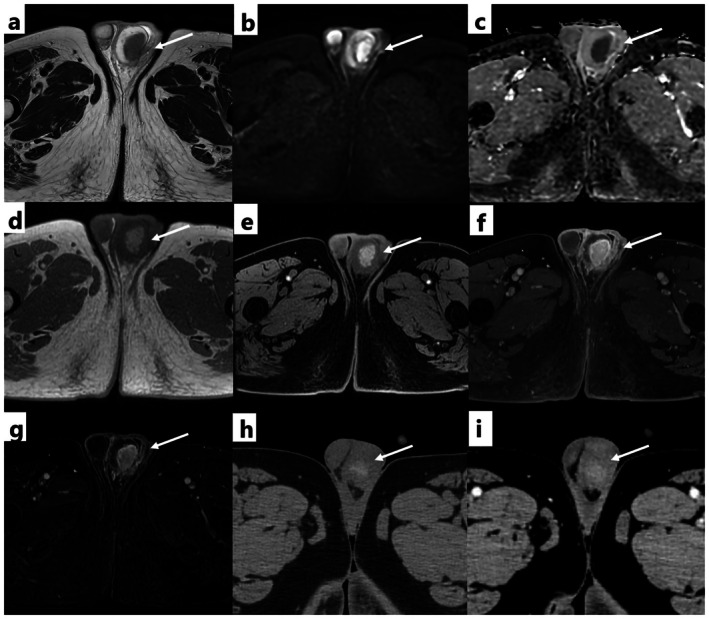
Magnetic resonance imaging (MRI) and computed tomography (CT) findings of the left testis. (a) T2‐weighed image, (b) diffusion‐weighted image (*b* = 1000 s/mm^2^), (c) ADC map, (d) T1‐weighed image, (e) fat‐suppressed T1‐weighed image, (f) contrast‐enhanced MRI (g) subtraction image (before and after contrast enhancement), (h) non‐contrast CT. (i) Contrast‐enhanced CT. MRI shows low signal intensity in the left testicular mass on T2WI (a; arrow), with diffusion restriction on diffusion‐weighted imaging (b; arrow) and the ADC map (c; arrow) with an ADC value of 0.84 × 10^−3^ mm^2^/s. T1WI (d; arrow) and fsT1WI (e; arrow) show high signal intensity and contrast enhancement is seen (d, e, f, and g; arrow). Non‐contrast CT (h) shows an enlarged left testis with a hyperattenuation area (arrow). Contrast‐enhanced CT (i) demonstrates only faint and indistinct enhancement of the lesion (arrow).
